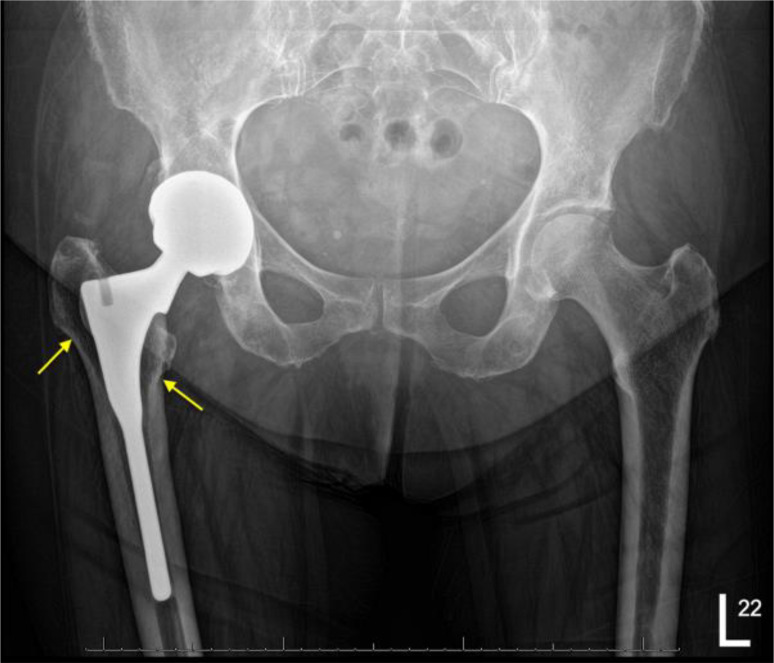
A 10-year post-operative radiograph with lucency at Gruen zones 1 and 7 (arrows) with no clinical signs or pain reported.
